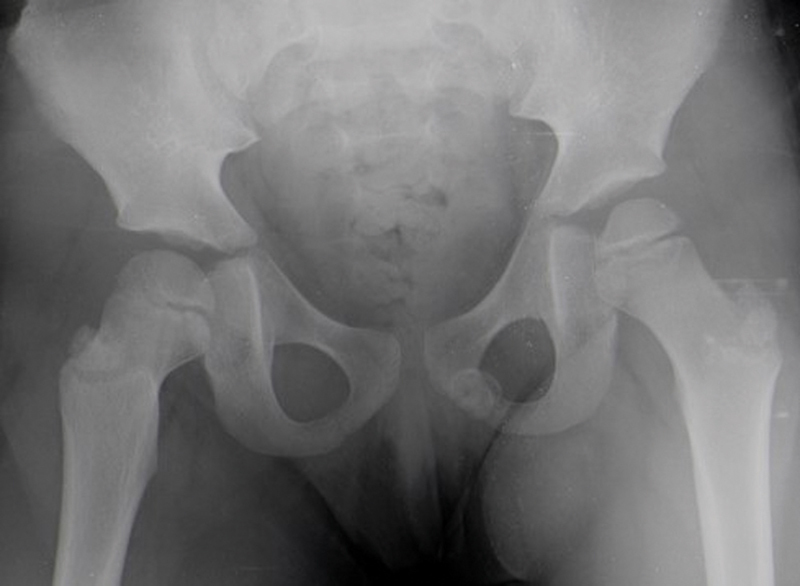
Radiography of the pelvis (anteroposterior view), revealing a radiopaque image at the level of the left ischiopubic branch, with well-defined contours.
